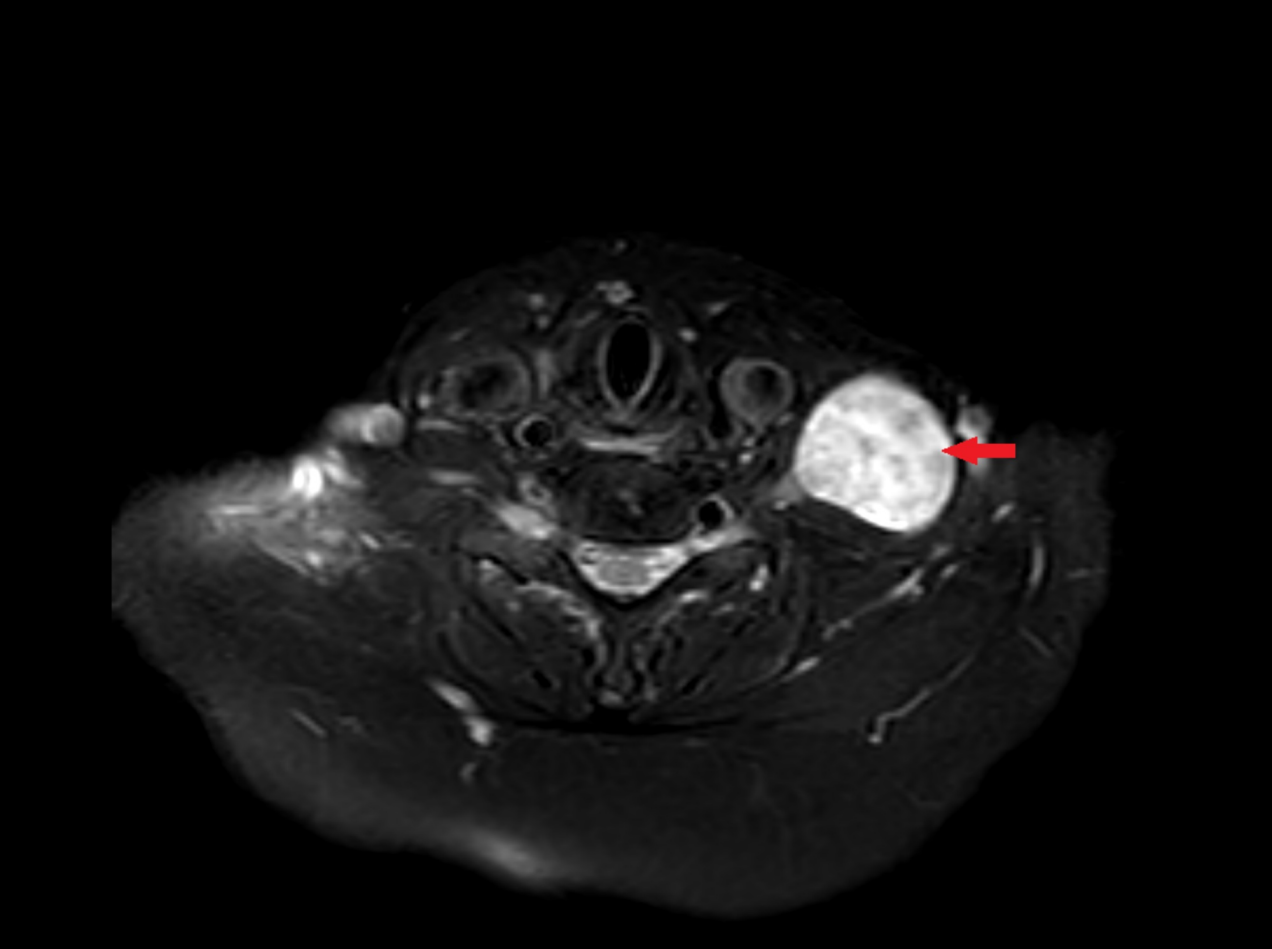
Axial T2 MRI of the neck A left-sided, well-circumscribed hyperintense neck mass (arrow) MRI, magnetic resonance imaging
